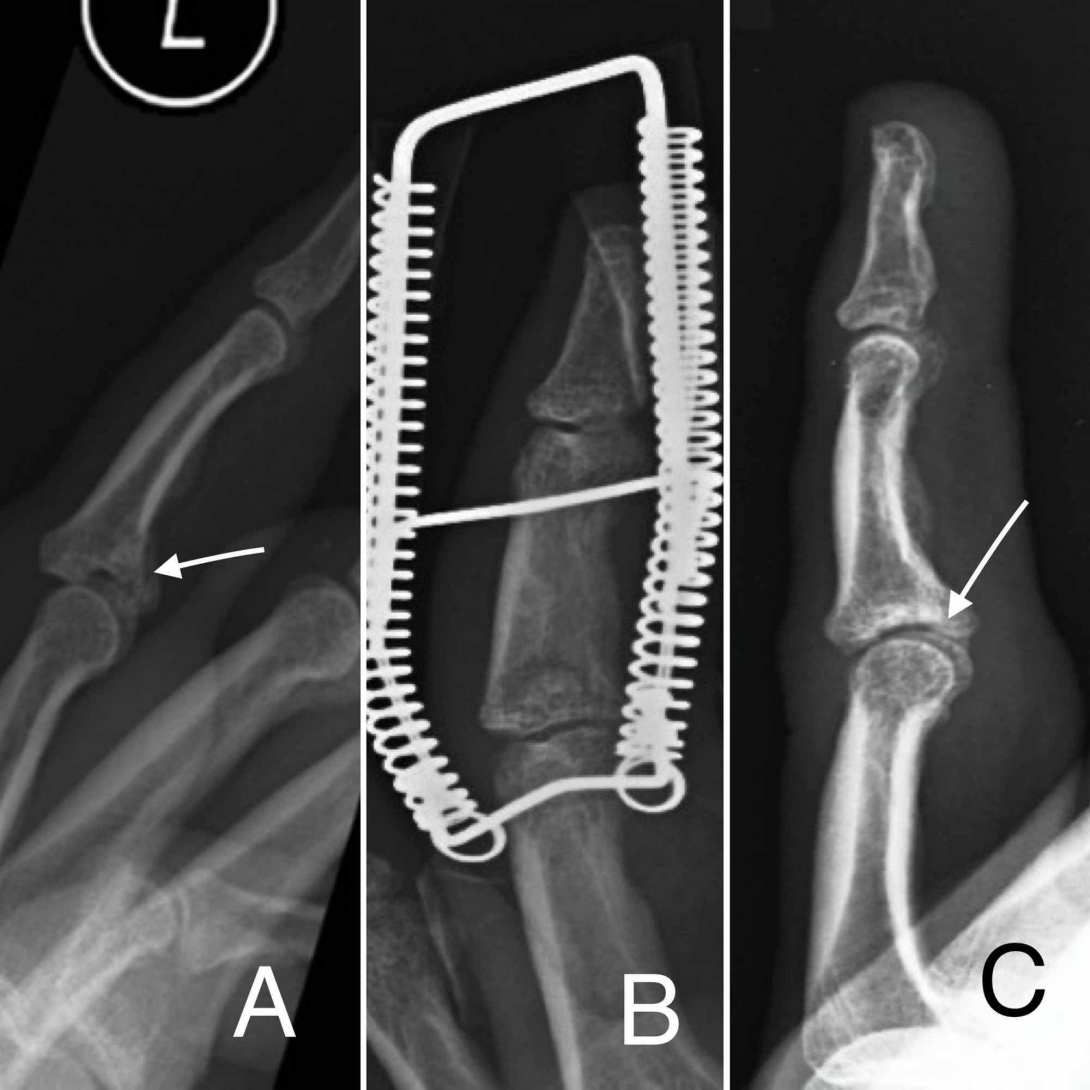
Radiographic images at various stages A: preoperative radiograph demonstrating E2 type fracture of the base of the middle phalanx left ring finger. B: two-week postoperative radiograph demonstrating fracture reduction using the Ligamentotaxor® device. C: six-month postoperative radiograph demonstrating fracture healing and restoration of joint congruency
